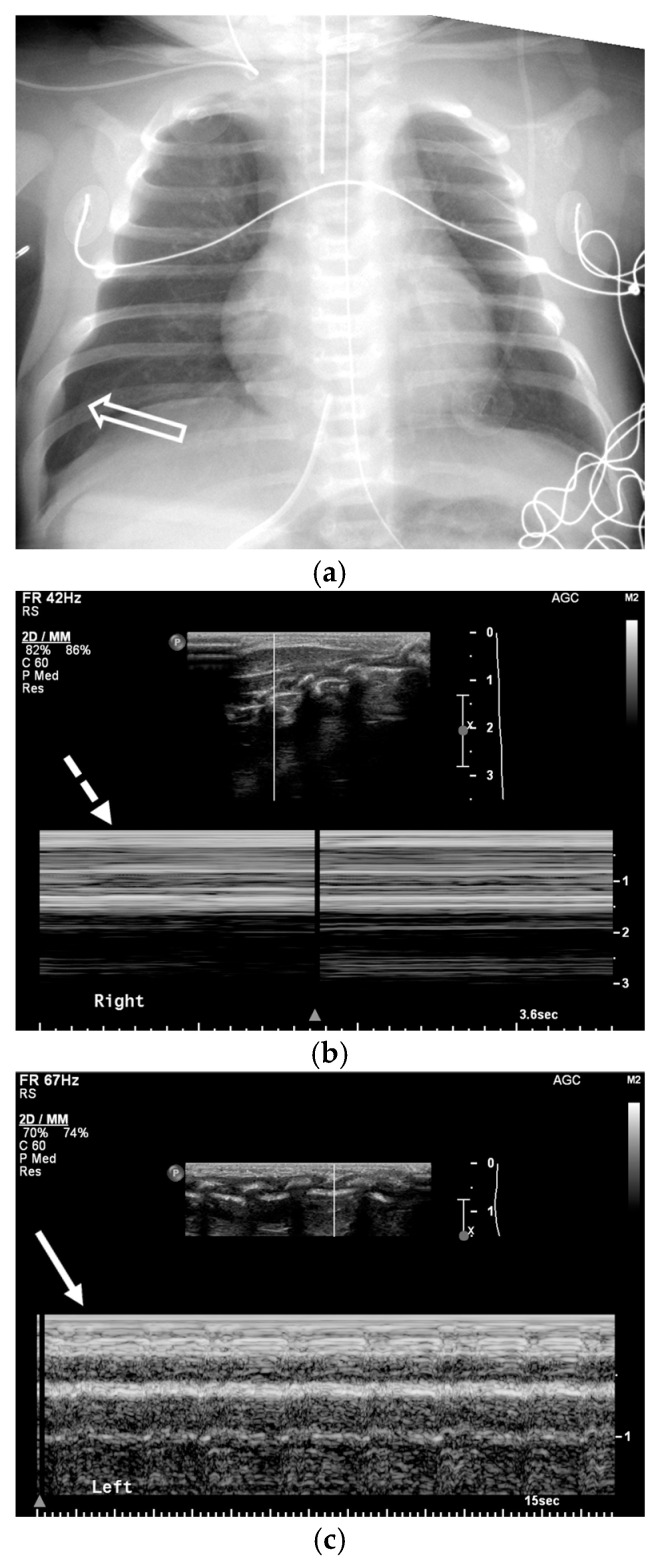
One-day-old neonate born at 39 weeks of gestation. Frontal chest radiograph (a) shows a small right pneumothorax (open white arrow). M-mode ultrasound image of right basilar hemithorax (b) reveals a barcode sign typical of pneumothorax (dashed white arrow). Comparison M-mode ultrasound image of left hemithorax (c) shows several normally expected B-lines and the presence of a seashore sign (solid white arrow).
